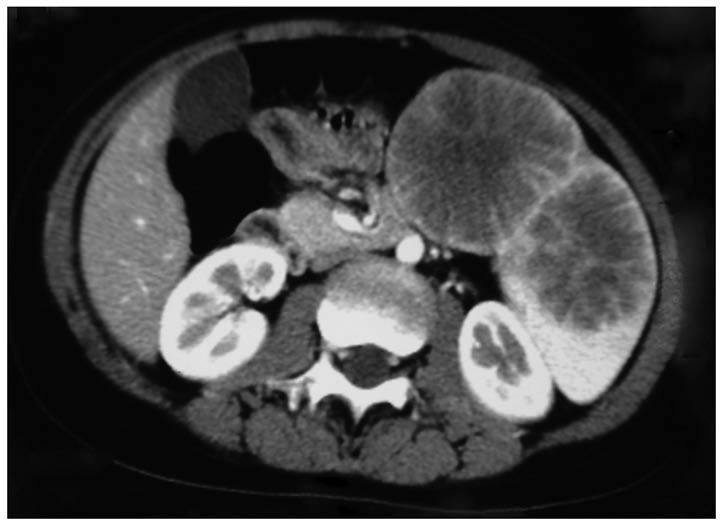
Computed tomography scan revealing two circular low density areas with clear boundaries. Circular, radiative, enhanced signals were observed at the margin of the tumor mass.
